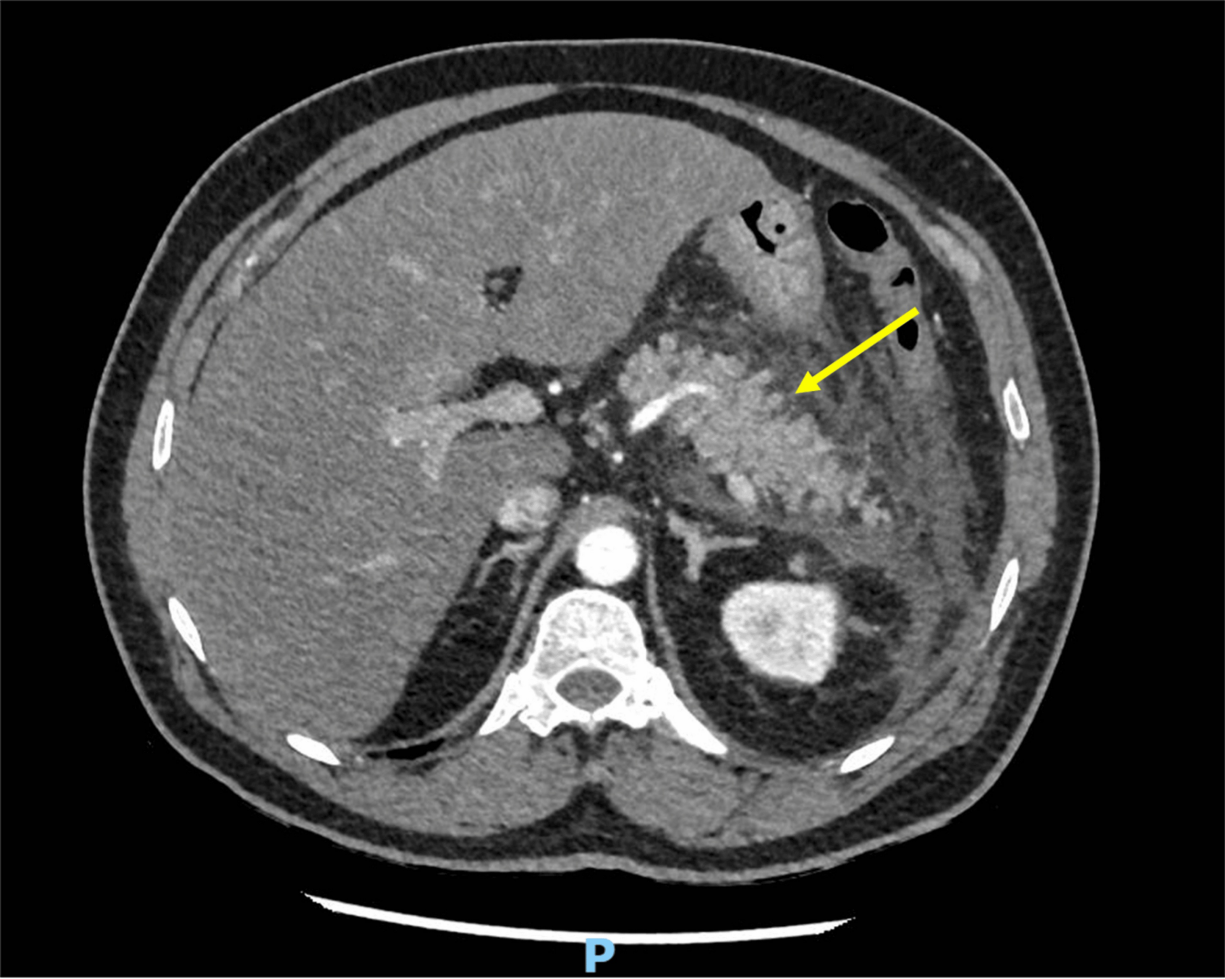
Abdominal CT scan axial view showing changes suggestive of acute pancreatitis (arrow) The Image shows bulky pancreatic body and tail with peripancreatic inflammatory changes.
